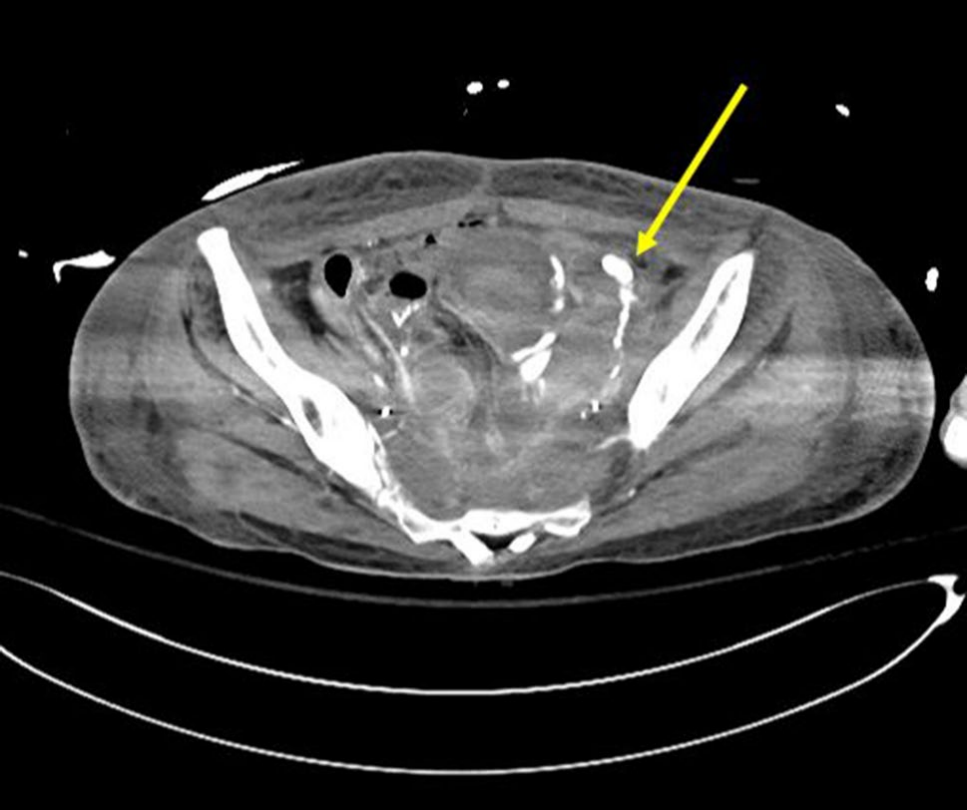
Contrast-enhanced CT angiography showing active extravasation in the pelvic cavity. The arrow indicates the site of contrast leakage, which was interpreted as evidence of ongoing hemorrhage from a vascular source. This finding guided the decision to proceed with embolization, suggesting the presence of an underlying entero-vascular fistula
